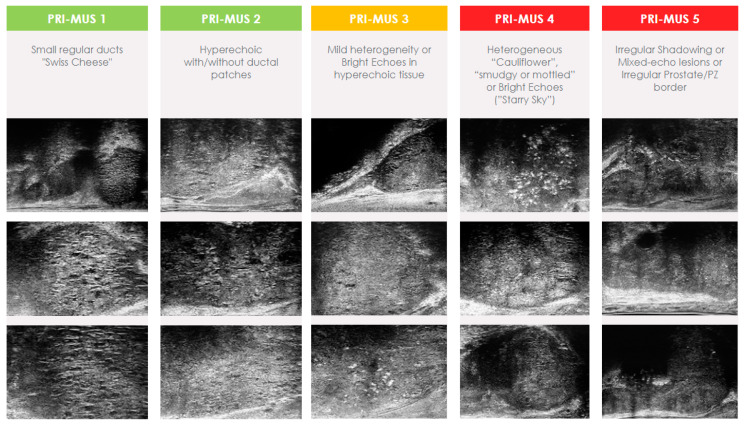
Prostate Risk Identification Using Micro-Ultrasound (PRI-MUS) score.
